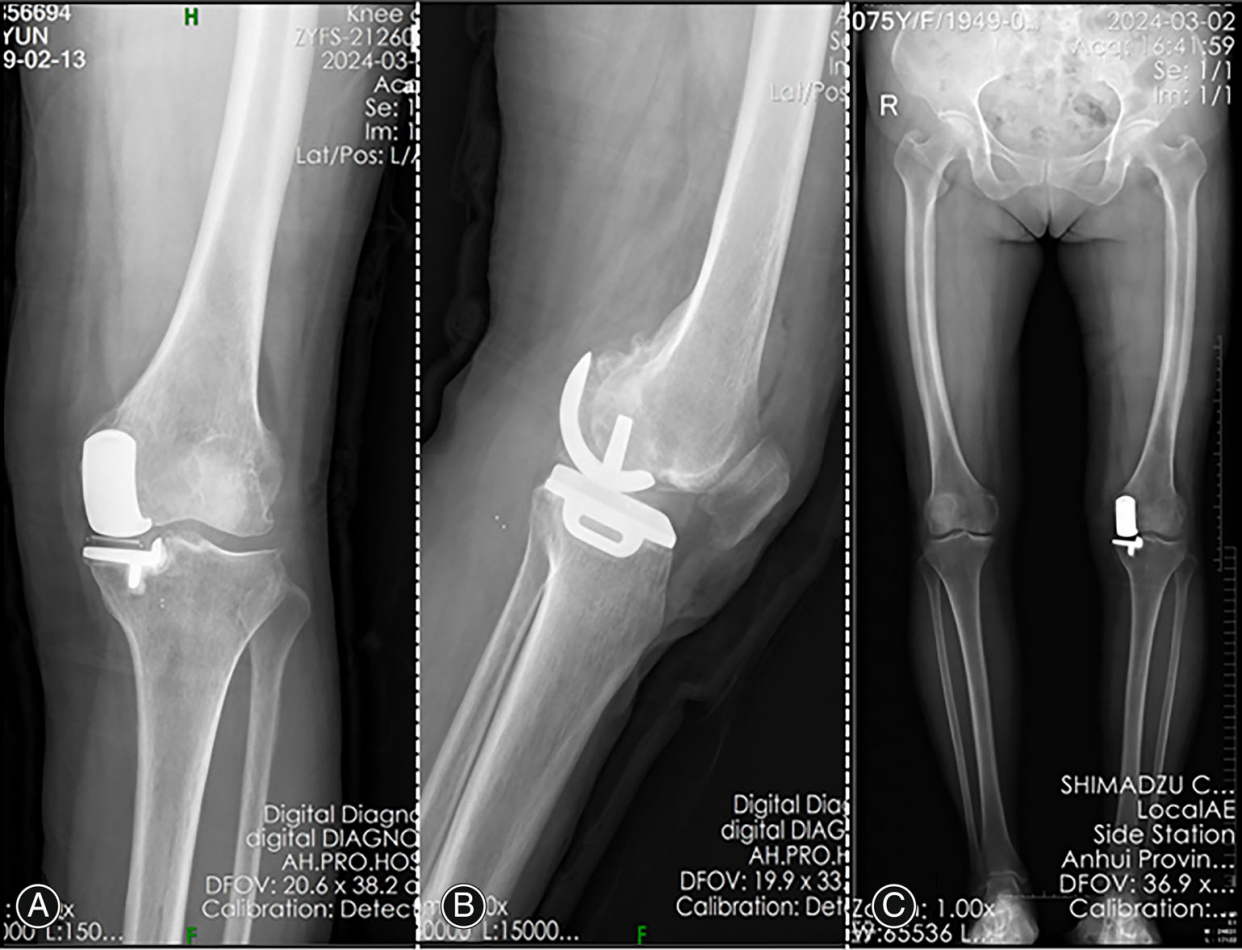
Postoperative radiographs showing replacement of the broken bearing with a 4‐mm thick mobile bearing, with the residual bearing fragment remaining in the left knee. (A) AP, (B) lateral, and (C) full‐length weight‐bearing of the lower extremities.
